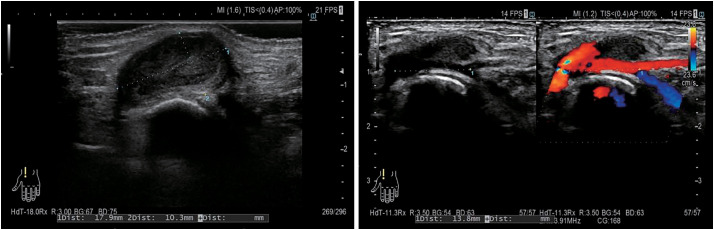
Preoperative ultrasound images. Preoperative ultrasound showing an 18 × 10 mm saccular aneurysm in the radial artery with pulsatile flow.
